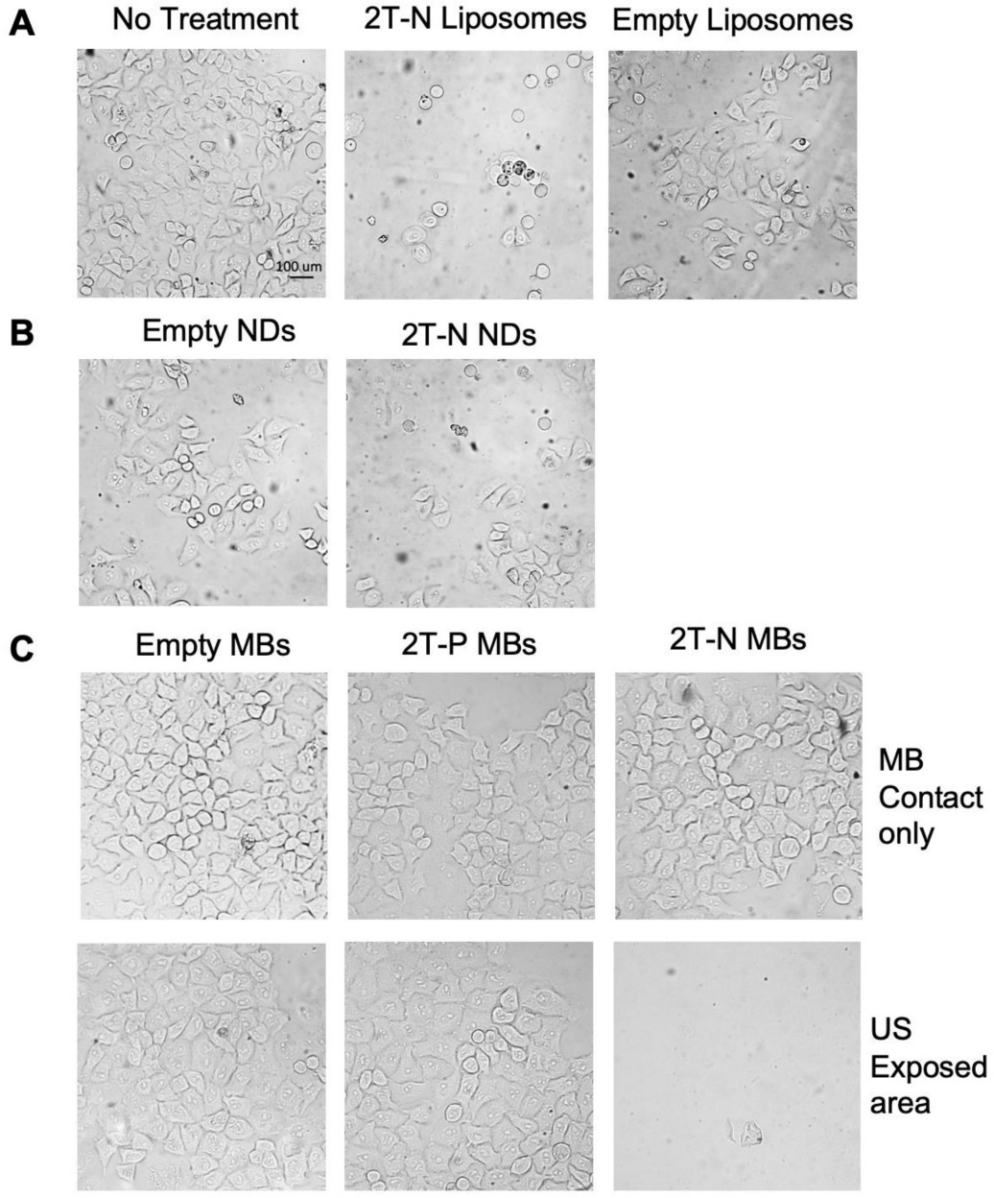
In vitro* prodrug loaded vehicle delivery. (A) Prodrug loaded liposome contact only, (B) ultrasound triggered nanodroplet delivery, (C) With and without ultrasound triggered delivery of prodrug loaded microbubbles.
